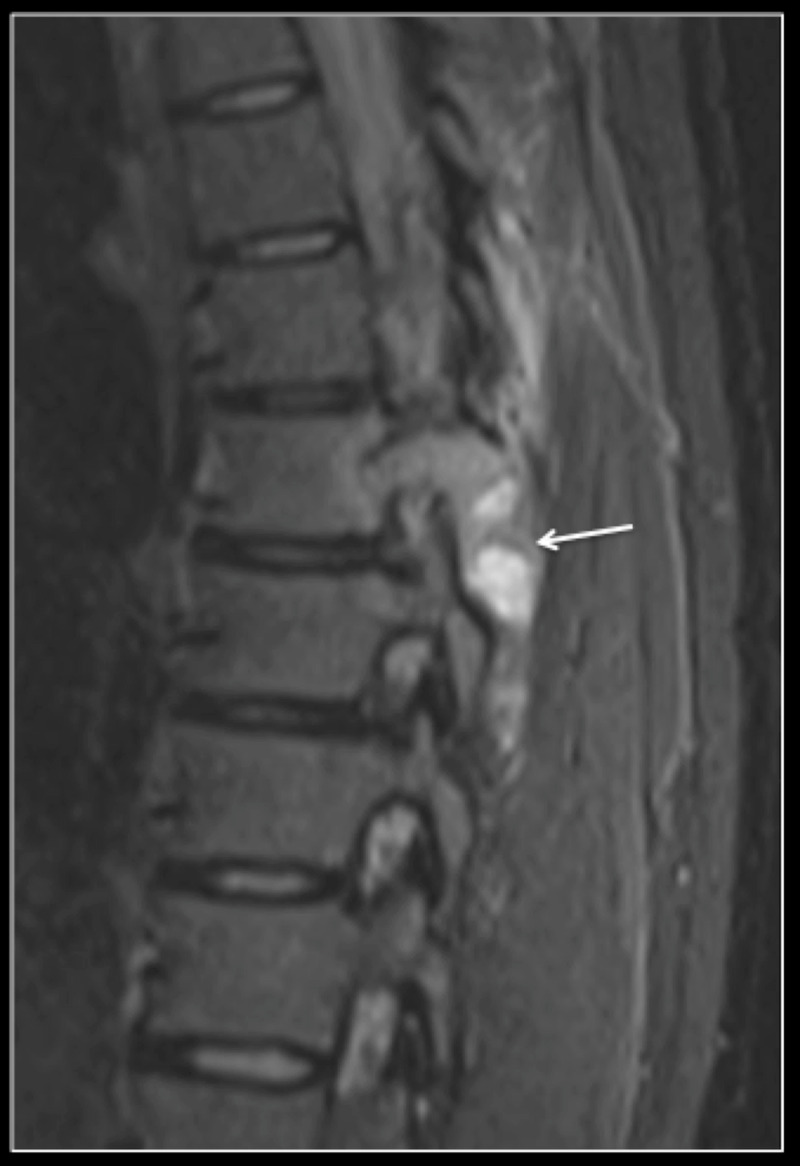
Sagittal STIR MRI of the thoracic spine showing a tumour (arrow) within the right pedicle and lamina with marked perilesional soft tissue oedema STIR: short-TI inversion recovery; MRI: magnetic resonance imaging
